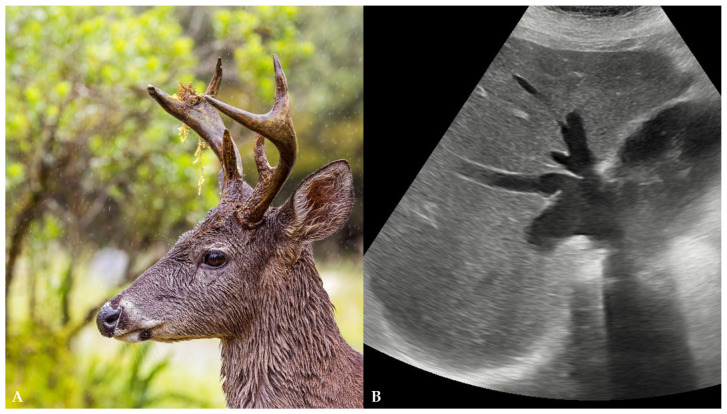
(A) An image of a white-tailed deer in Chingaza National Park, Colombia [author Charles J. Sharp; available at https://commons.wikimedia.org/wiki/File:White-tailed_deer_(Odocoileus_virginianus_goudotii)_male_head_Chingaza.jpg (accessed on 24 March 2025)]. (B) An ultrasound image of the deer horn sign where the dilated intrahepatic inferior vena cava represents the deer’s head and dilated hepatic veins represent the deer’s horns.
